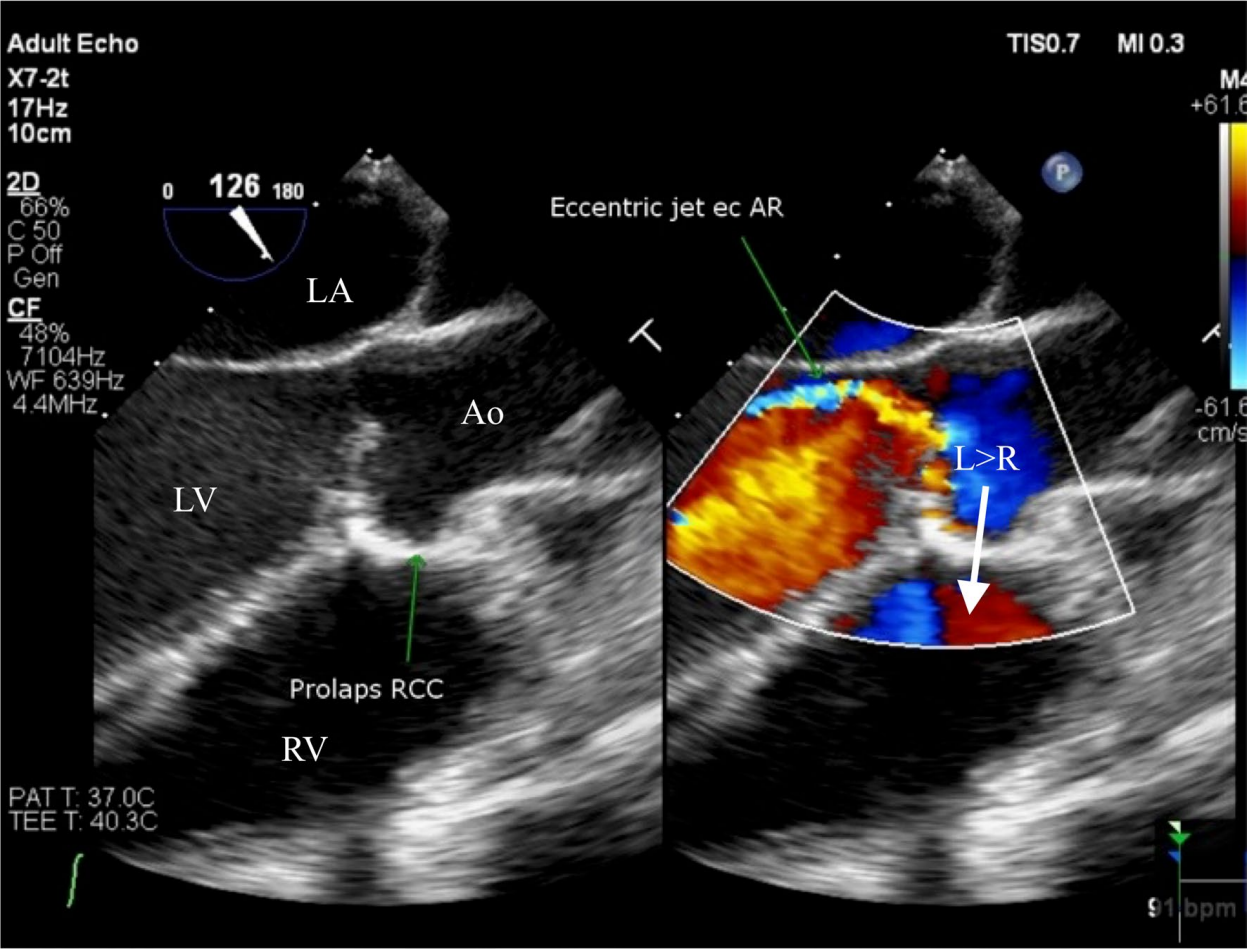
Transoesophageal echocardiography showed RCC Prolapse caused moderate AR. Ao = aorta; RV = right ventricle; LV = left ventricle; LA = left atrium; RCC = right coronary cusp; L = left; r = Right
